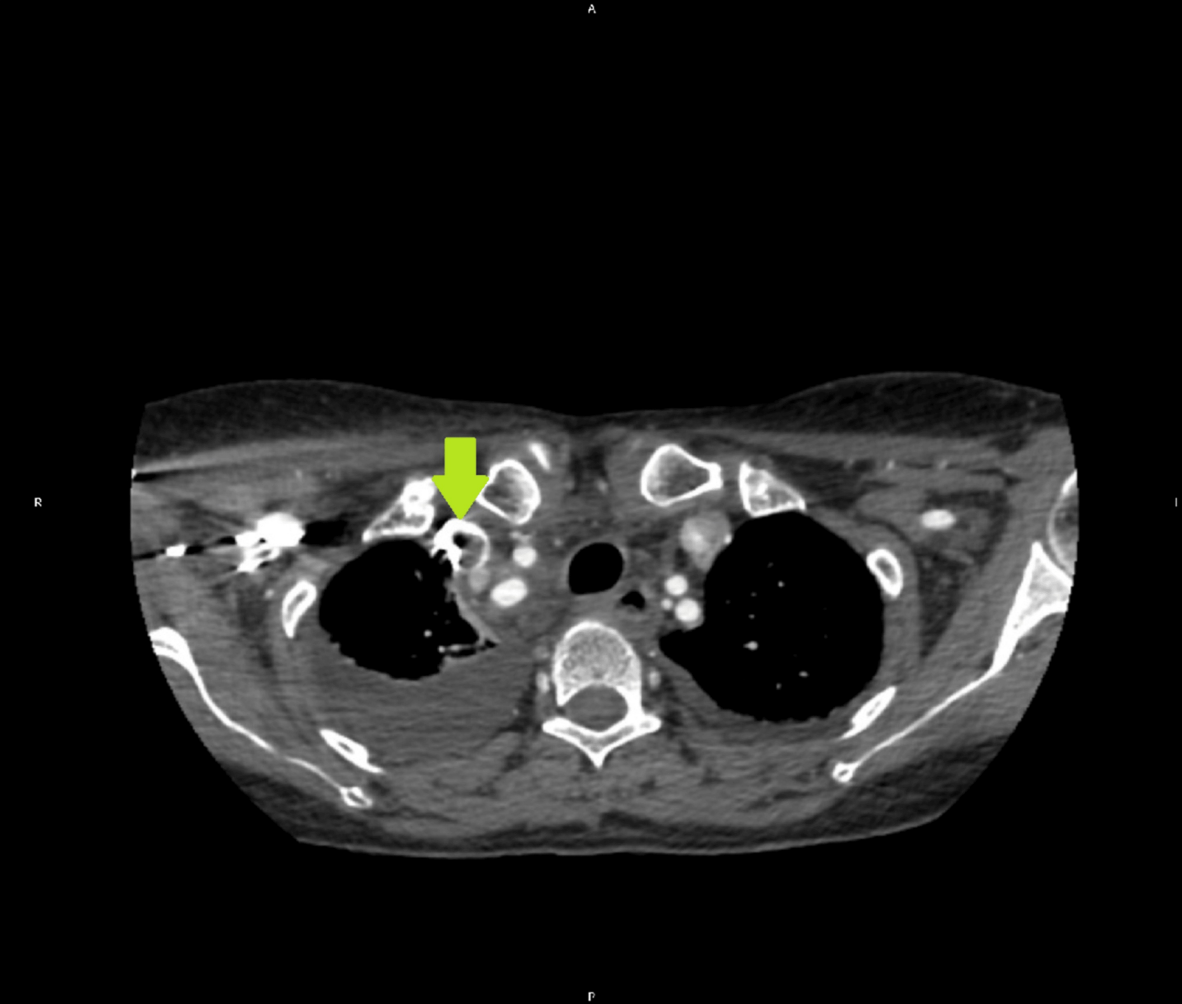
CT angiogram confirming the presence of intraluminal thrombus (arrow).
